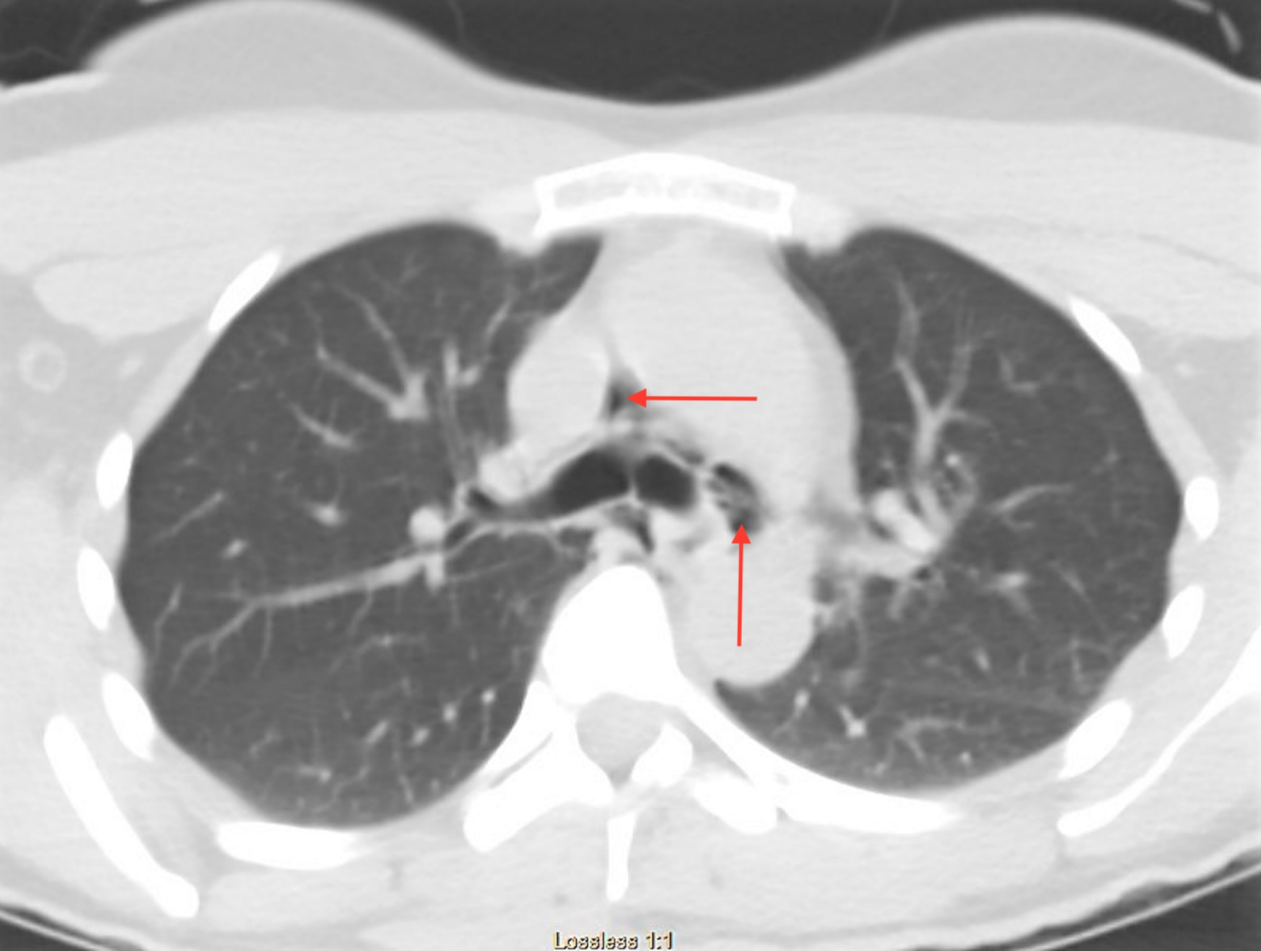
Axial CT of the thorax with air seen around the major bronchi.
